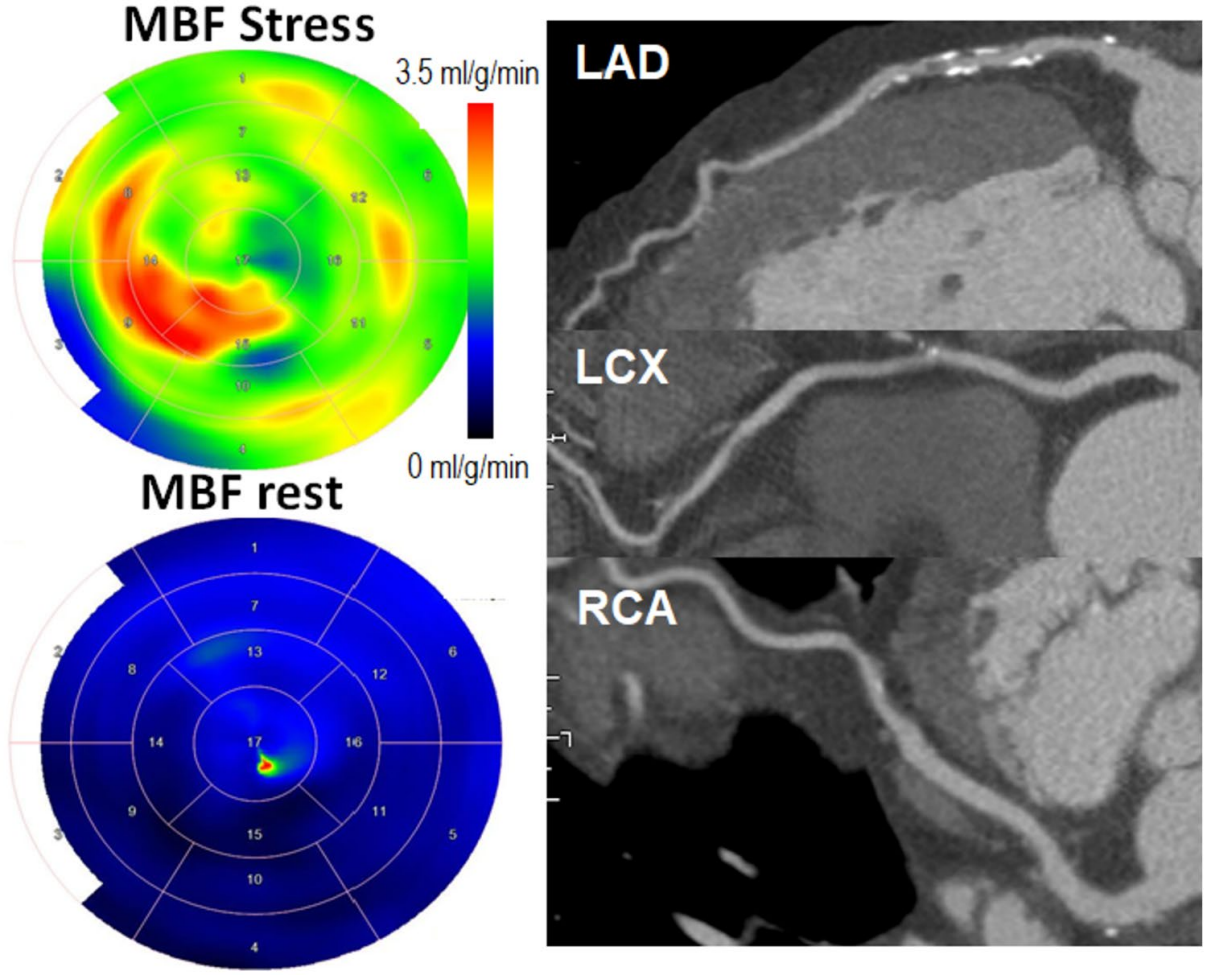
Coronary CT angiography and positron emission tomographic (PET) myocardial perfusion images in a 62-year-old man with atypical chest pain, diabetes, dyslipidemia, and hypertension. Coronary artery calcium (CAC) scan showed moderate CAC score of 210. Coronary CT angiography showed partially calcified atherosclerosis in the left anterior descending, left circumflex, and right coronary arteries (LAD, LCX, and RCA, respectively). Maps of myocardial blood flow (MBF) measured by ^15^O-water PET showed no significant myocardial ischemia and invasive coronary angiography showed no obstructive CAD. However, based on PET, coronary flow reserve was impaired (1.8), suggesting coronary microvascular dysfunction
